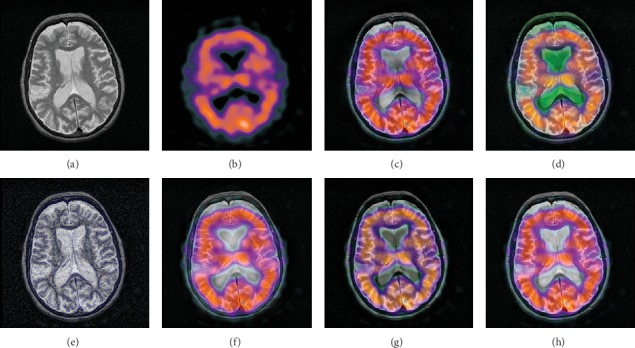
MR and SPECT medical image fusion results. (a) MR original image. (b) SPECT original image. (c) NSST-PAPCNN. (d) CSR. (e) MST-SR. (f) NSCT-SR-PCNN. (g) SR-PCNN. (h) Proposed.
